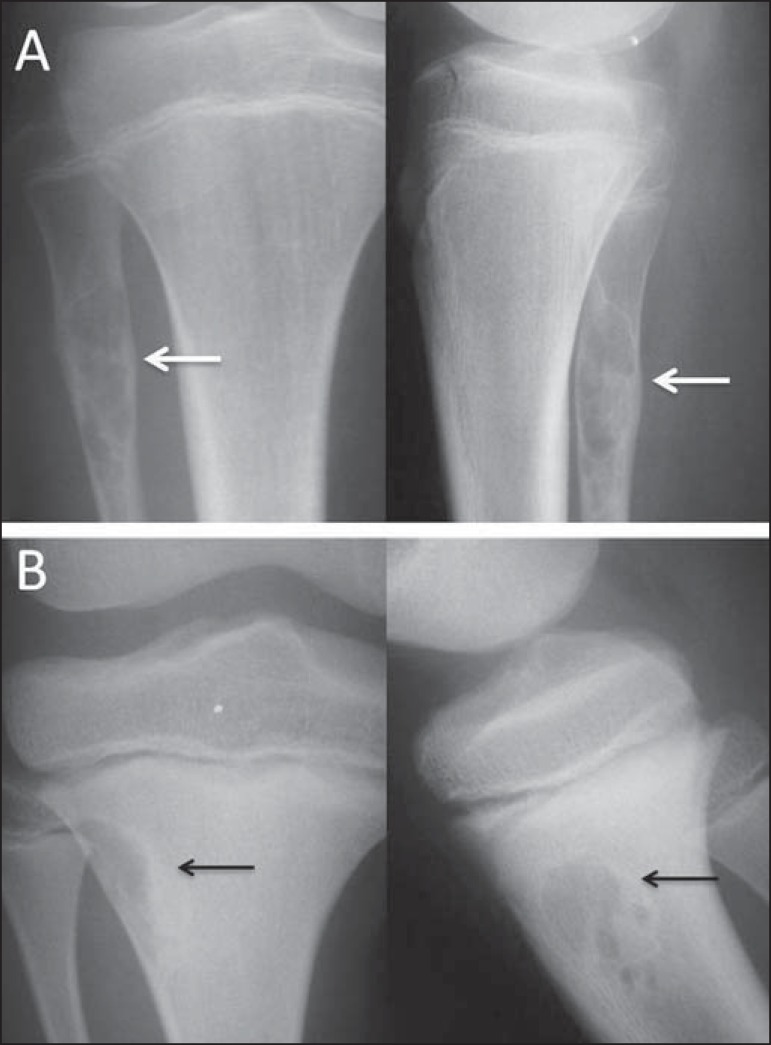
A: Anteroposterior and lateral radiography of an
